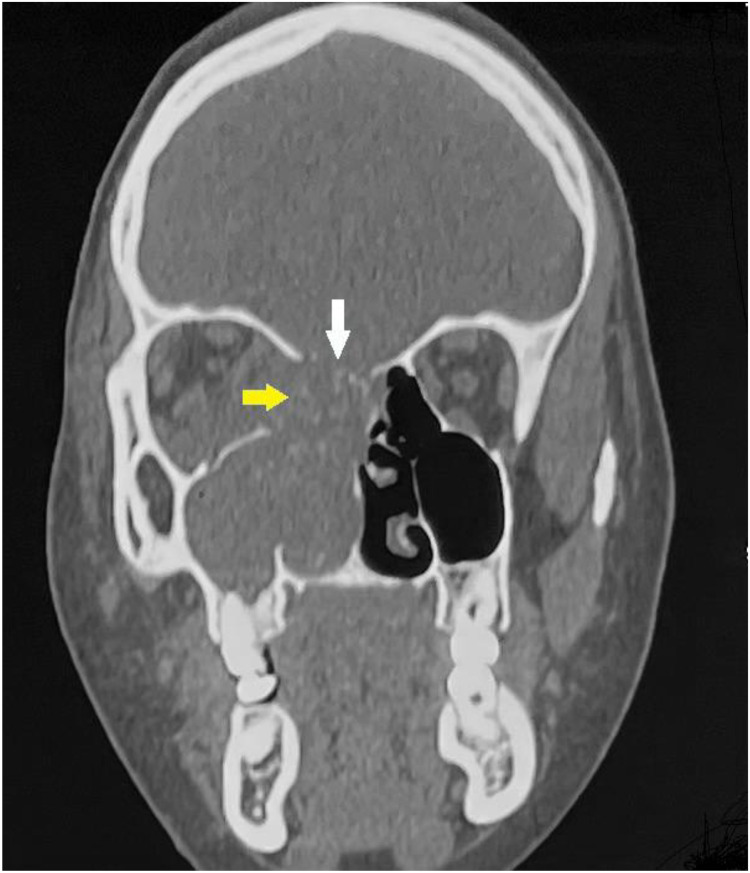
Coronal bone-window contrast-enhanced head and neck CT demonstrates a right ethmoido-maxillo-nasal mass exhibiting internal calcifications and completely occupying the ipsilateral nasal cavity. The lesion demonstrates focal osseous destruction of the lamina papyracea (yellow arrow) and erosion of the right ethmoidal roof (white arrow).
